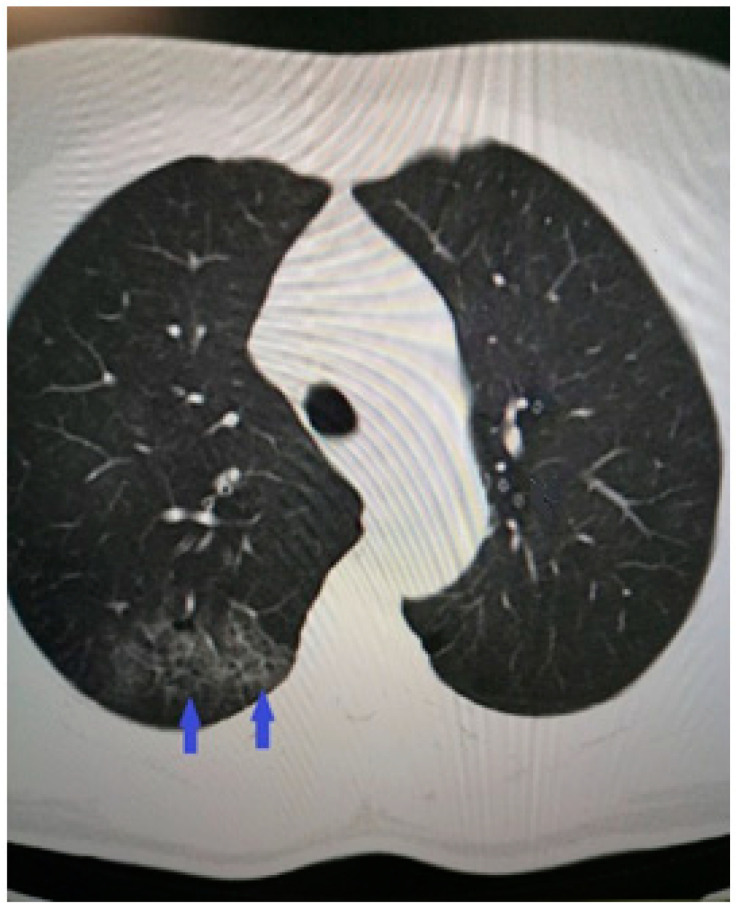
Chest CT showing peripheral right-sided consolidations and parenchymal ground-glass opacities consistent with COVID-19 pneumonia.
